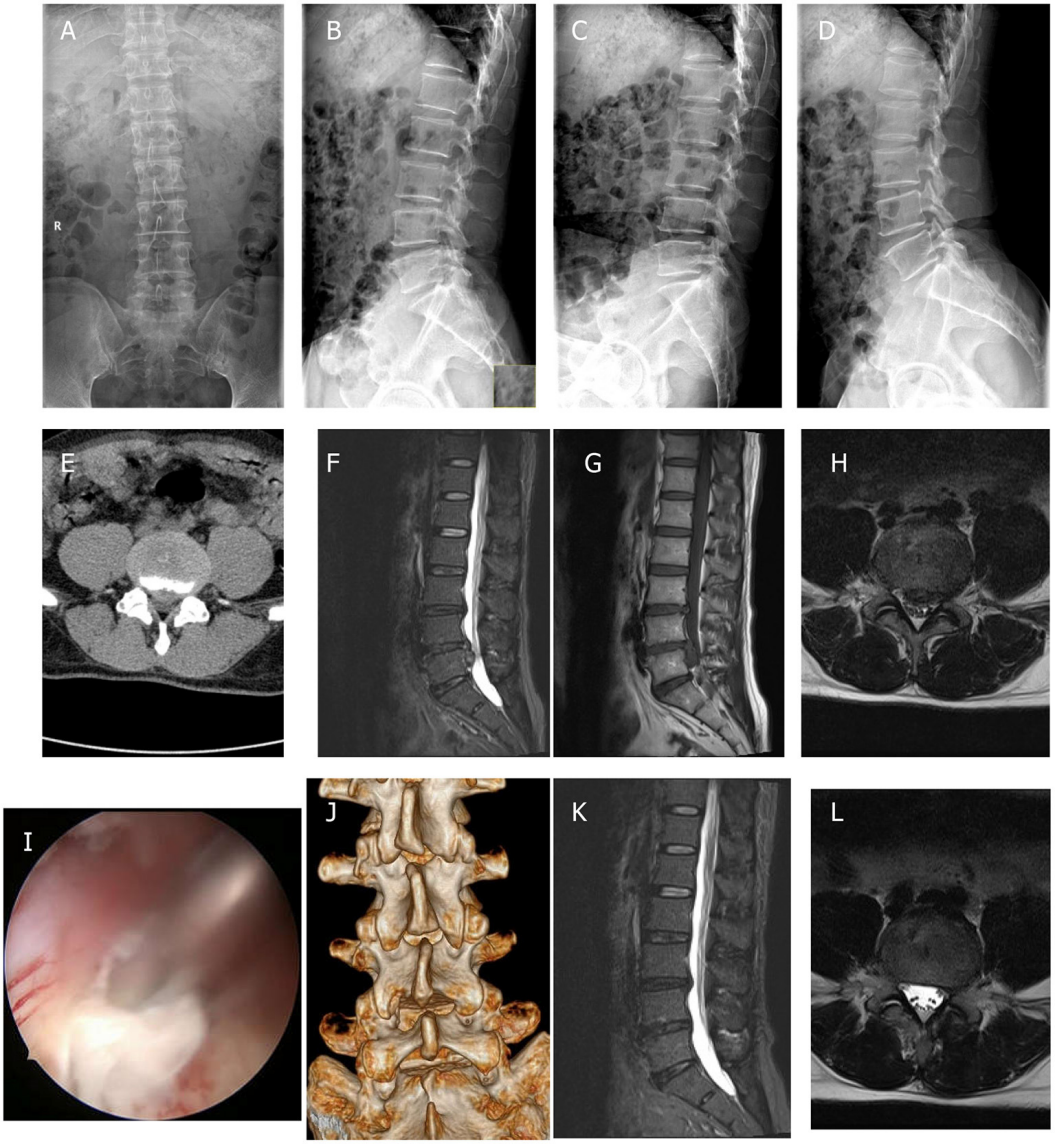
Unilateral biportal endoscopic (UBE) diskectomy was performed on a 47-year-old male patient with L4/5 lumbar disc herniation. (A,B) Preoperative anteroposterior and lateral plain radiographs; (C,D) preoperative flexion and extension radiographs; (E) preoperative CT scans; (F–H) preoperative MRI scans; (I) diskectomy was performed to ensure adequate decompression of the nerve tissue; (J) postoperative 3D-CT scans; and (K,L) postoperative MRI scans.
